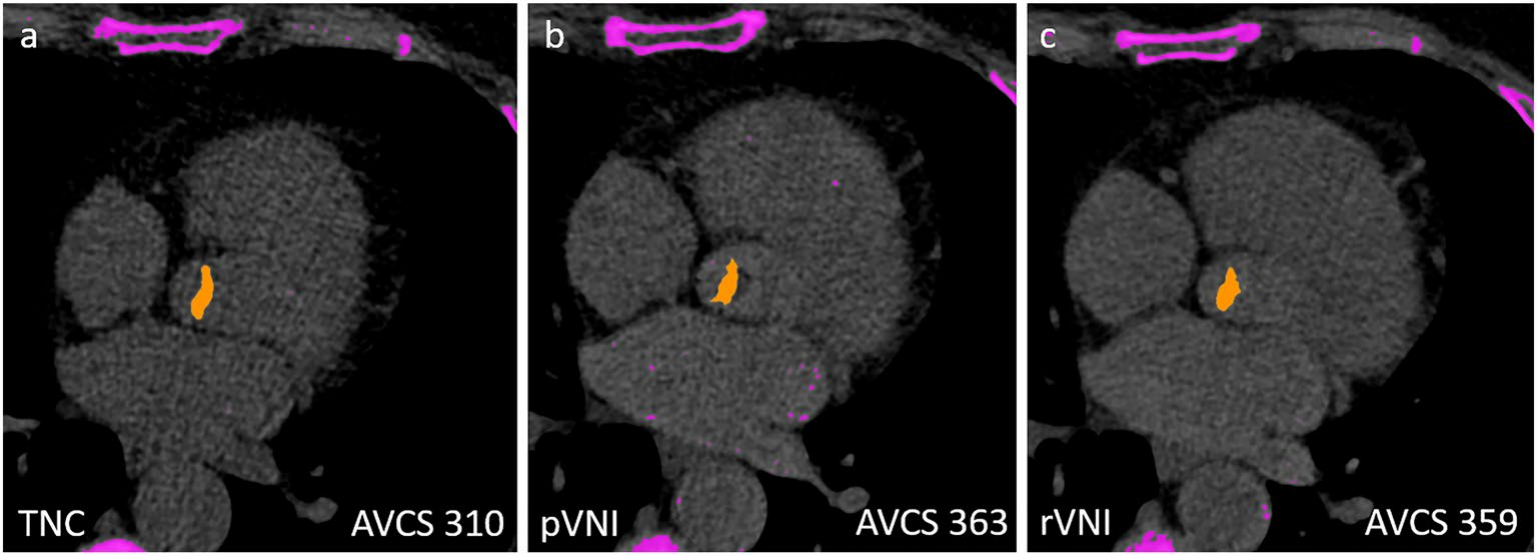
True non-contrast (TNC) CT (a), prospective virtual non-iodine (pVNI) CT (b), and retrospective virtual non-iodine (rVNI) CT (c) in a 62-year old woman demonstrate high concordance of aortic valve calcification score (AVCS) across all three calcium-scoring approaches.
